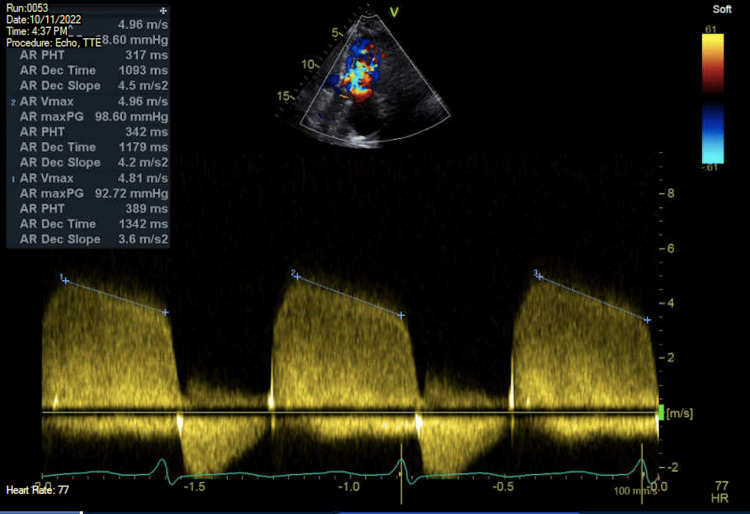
Two-chamber transthoracic echocardiogram view with Doppler evaluating regurgitant flow across the aortic valve
